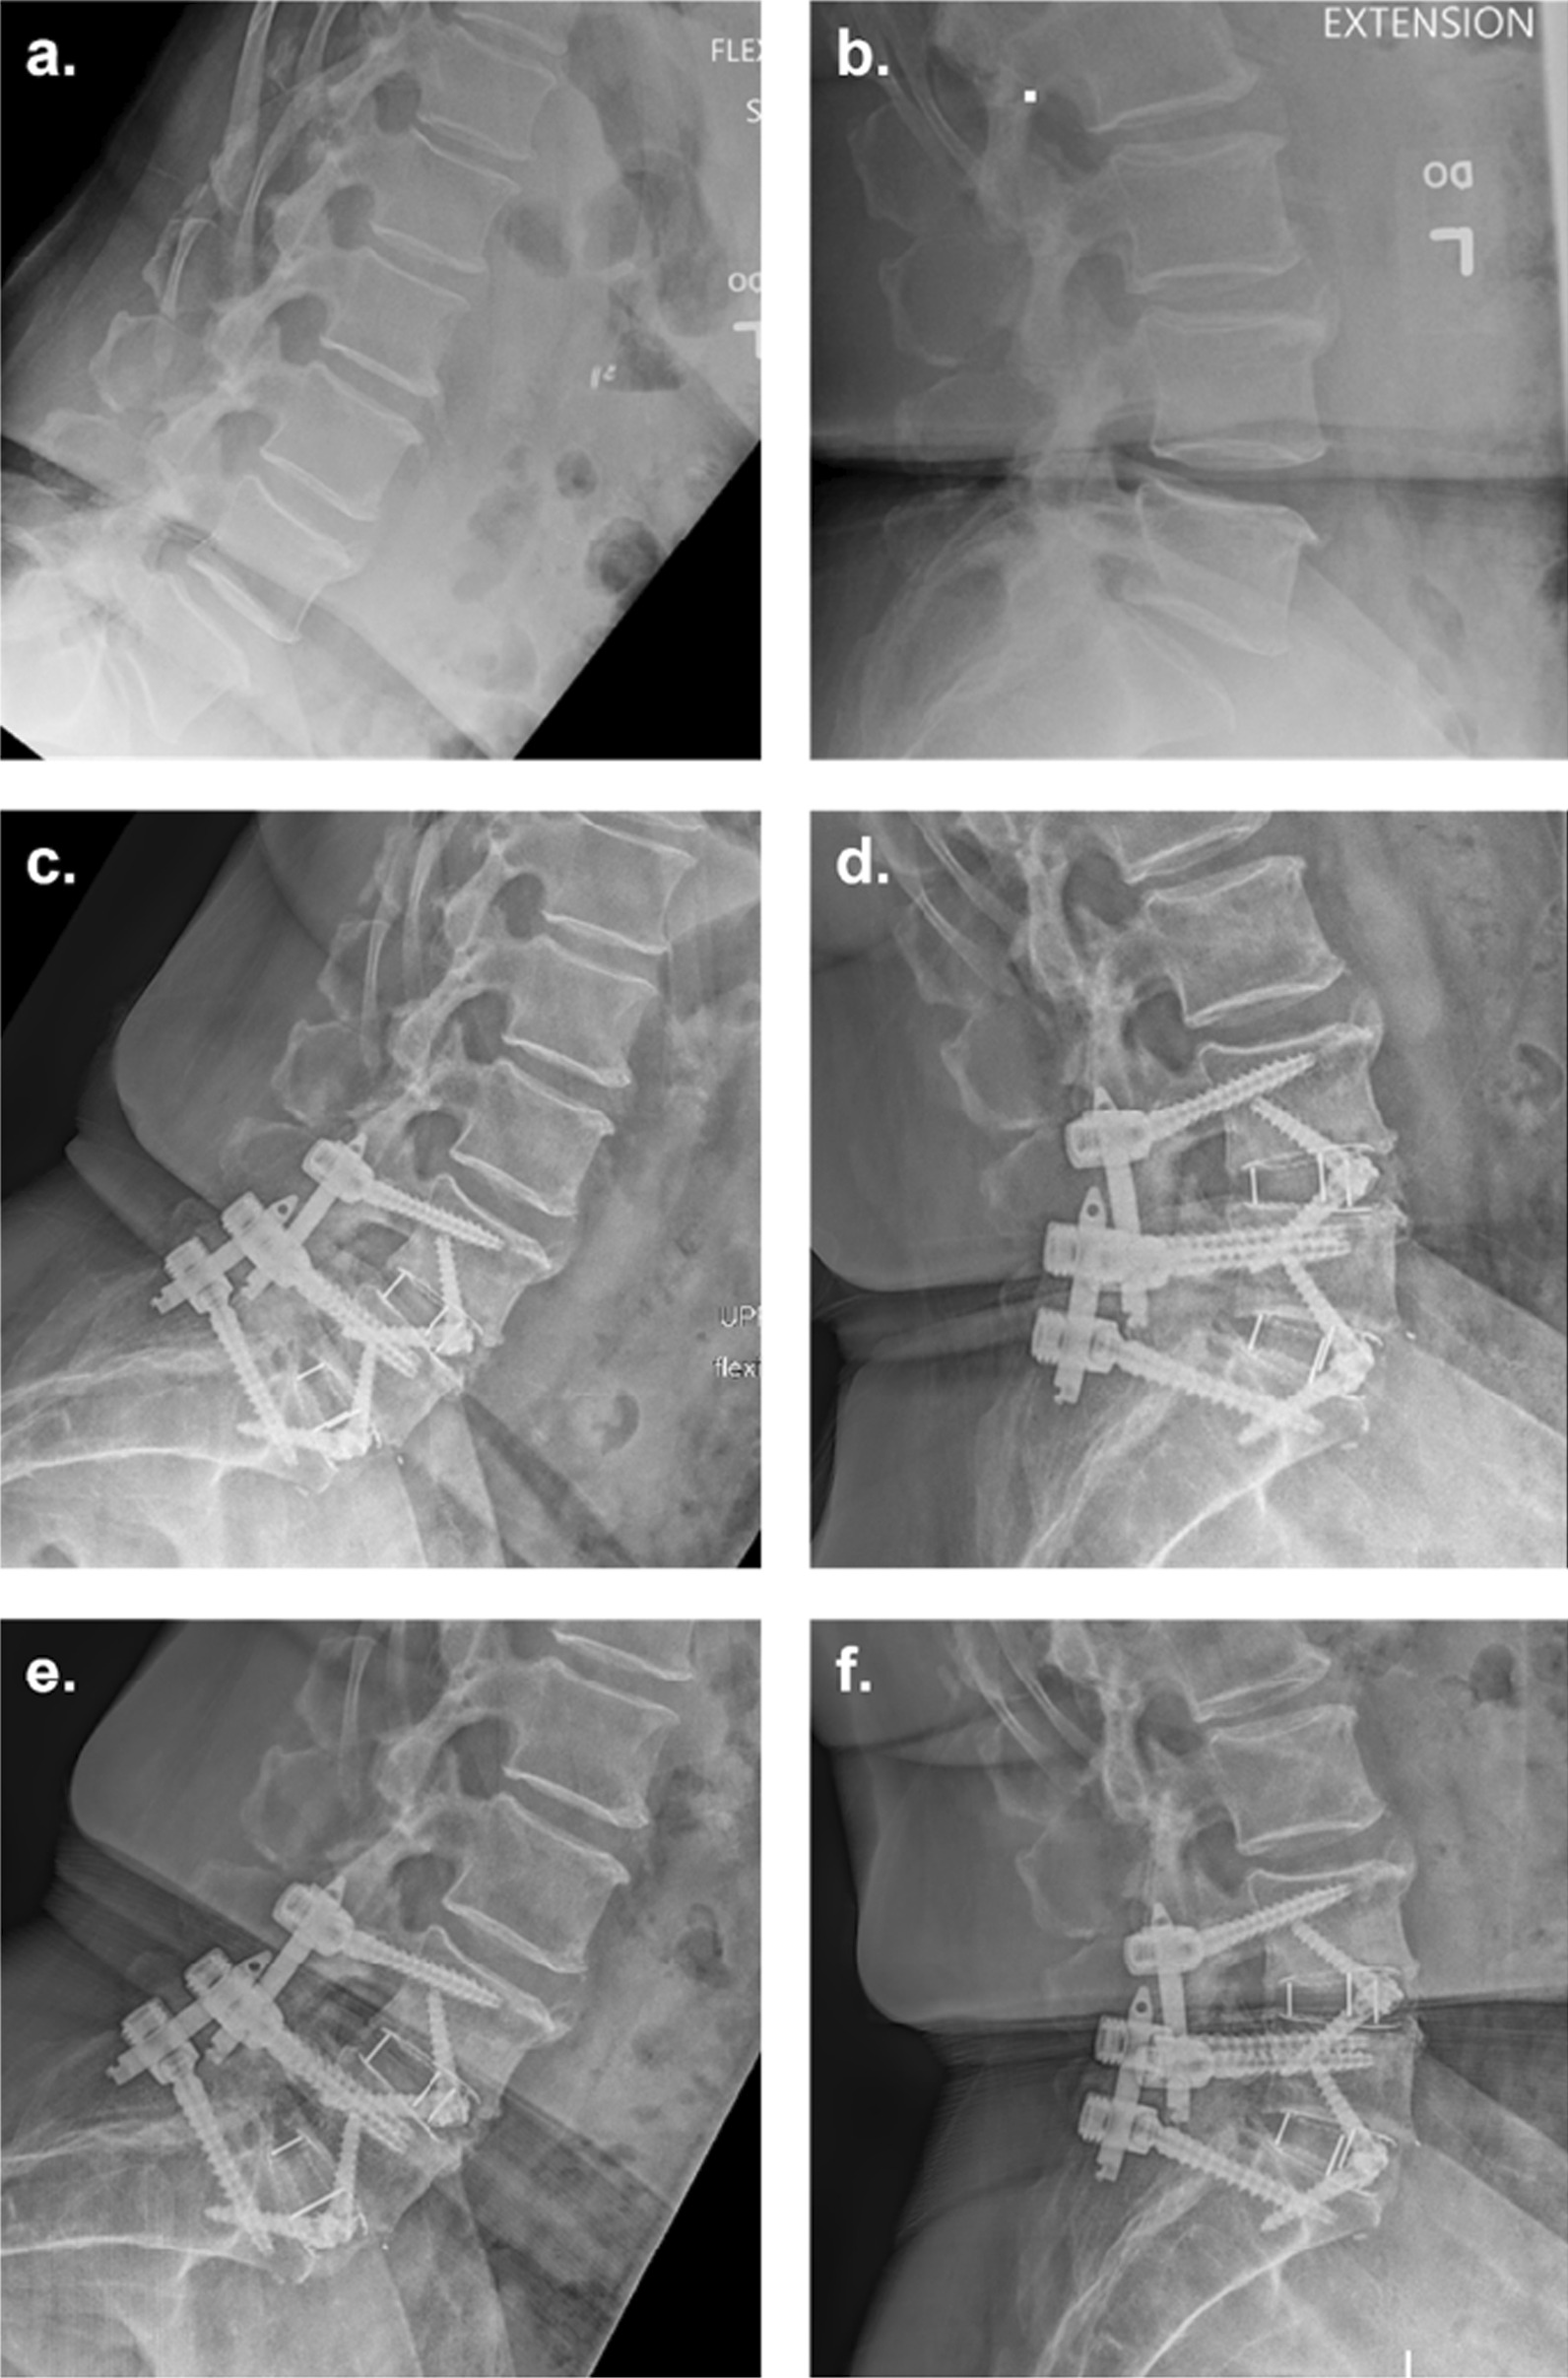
Pre- and post-operative radiographs of a 2-level ALIF using ViBone on a 74-year-old overweight female at L4–L5 and L5–S1. a Pre-operative flexion radiograph b Pre-operative extension radiograph c six-month flexion radiograph d six-month extension radiograph e Twelve-month flexion radiograph f Twelve-month extension radiograph
